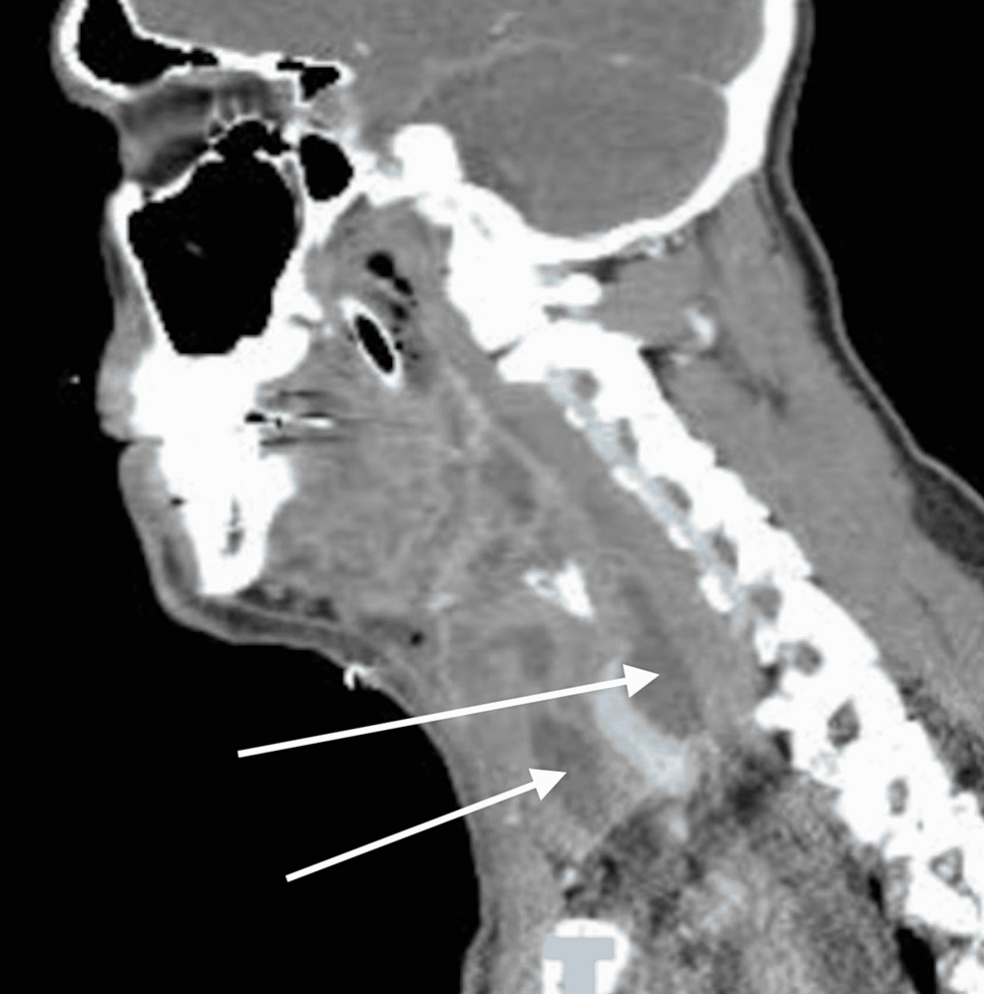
Sagittal view of the CT scan on hospital day 5 White arrows represent fluid collection from descending infection.
